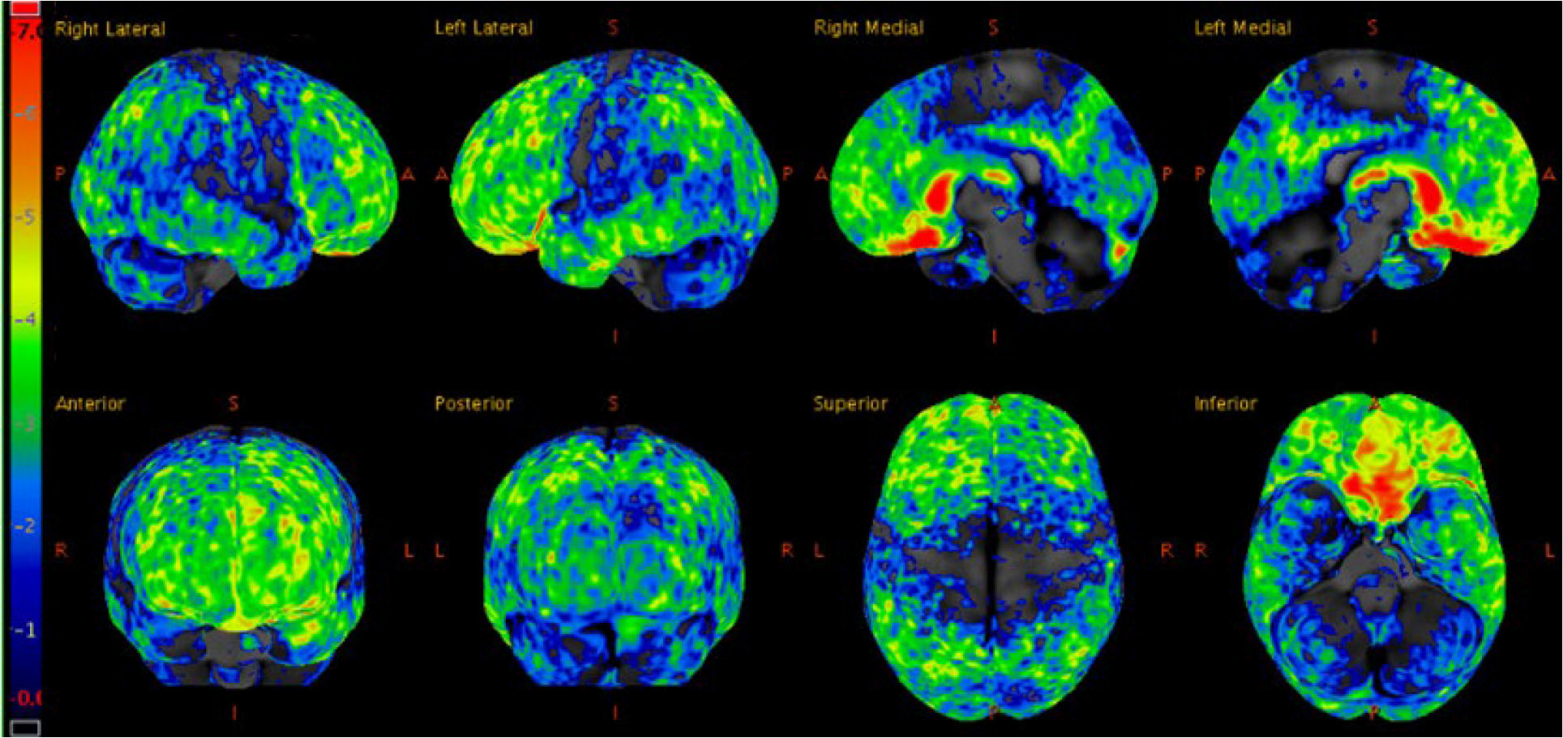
Statistical z-score maps of brain FDG-PET at age 27, with the degree of hypometabolism reflected by the color bar on the left; dark blue is within normal limits and red is maximally abnormal. Note the degree of hypometabolism is severe in the orbitofrontal and caudate regions, moderate in the frontotemporal regions (left slightly more so than right), and mild to moderate in the parietal and posterior cingulate regions.
